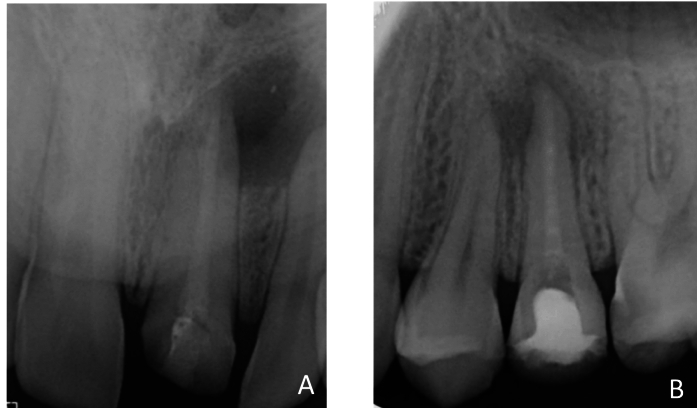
Periapical radiographs were taken in patients to assess the placement of intracanal dressing material. A Calcium silicate-based intracanal dressing, B calcium hydroxide-based intracanal dressing
